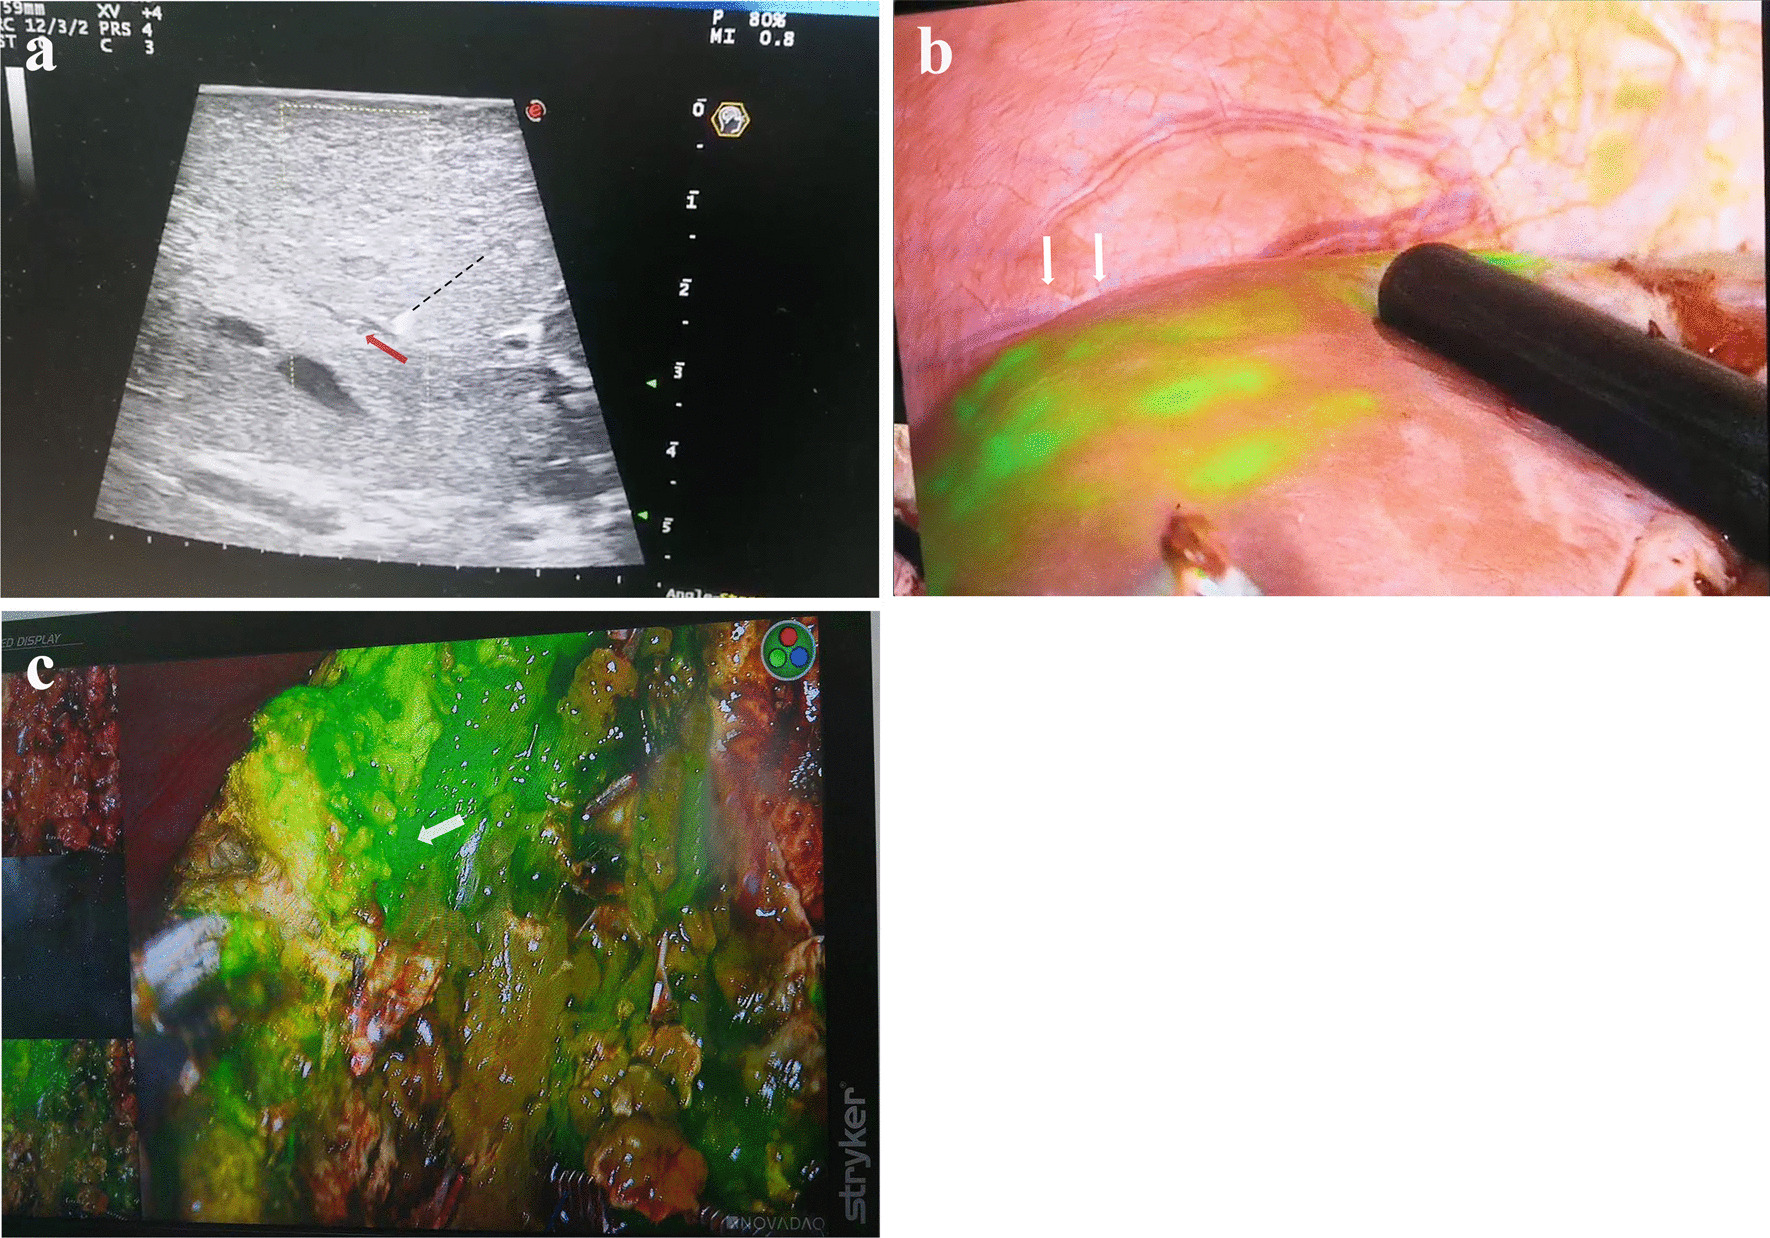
a Portal vein branch of the target hepatic segment was super-selected and punctured with a 18G needle by intraoperative laparoscopic ultrasound guidance (black dotted line), then an injection of 0.05 mg indocyanine green was performed gently (0.025 mg/ml, red arrow); b Visualization of the lesion area by fluorescence imaging after the intraoperative portal vein injection of indocyanine green (white arrow); c Real-time indocyanine green fluorescence imaging (ICG-FI) enabled the visualization of overall structure of the lesion and intrahepatic surgical boundary during operation (white arrow)
